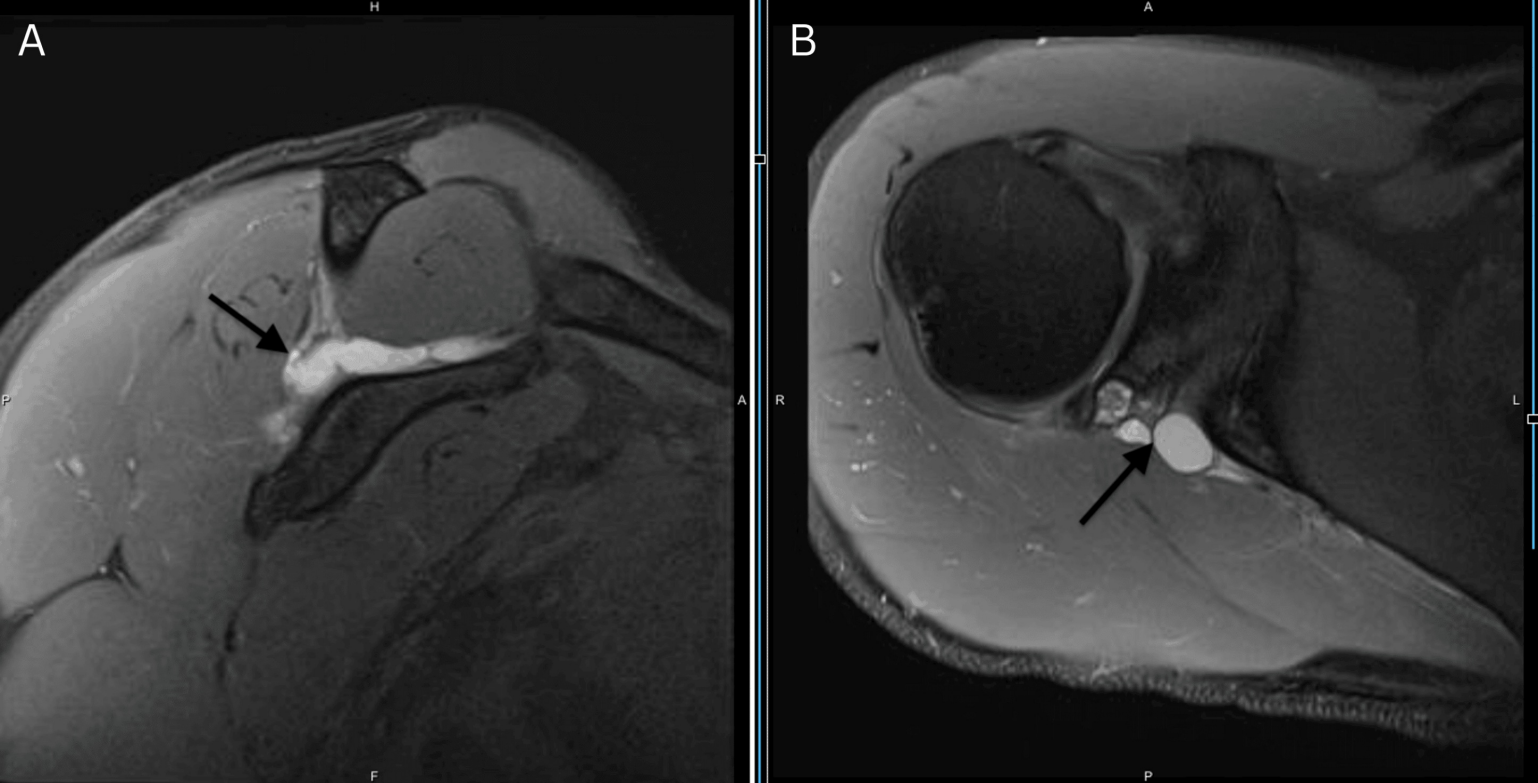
Pre-aspiration MRI image: MRI image showing a paralabral cyst compressing the suprascapular nerve (A) Pre-aspiration coronal MRI image showing a paralabral cyst compressing the suprascapular nerve. An arrow pointing at fluid collection causes nerve impingement. (B) A pre-aspiration axial MRI image shows a paralabral cyst compressing the suprascapular nerve.
